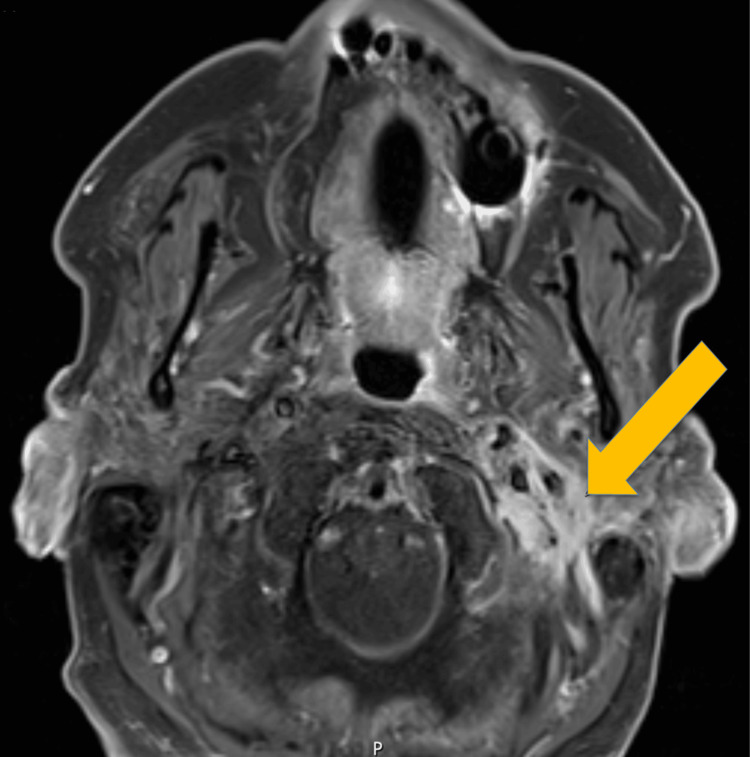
MRI of the brain, axial section, T1, fat saturation with contrast, showing complete opacification of left mastoid air cells with erosions of the mastoid septae and enhancing debris throughout the left mastoid cavity extending into the left middle ear.
